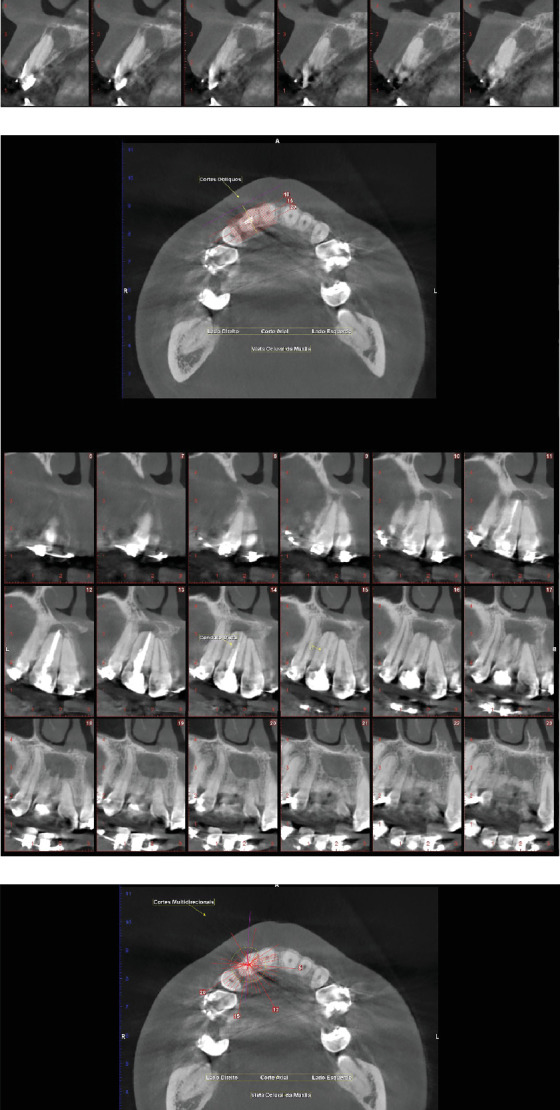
(a–c) Computed tomography showing an overall view of the lesion, canal anatomy, mineralized tissue isthmus, and bifurcation in the third apical. Transaxial, oblique, and multidirectional cuts of the region of Tooth 12 for visualization of the bone and dental structures. Tooth 12 shows a longitudinal hypodense (radiolucent) image on the distal surface of the root, suggestive of an untreated distal root canal, as well as a unilocular, expansive hypodense lesion located in the apical region.
